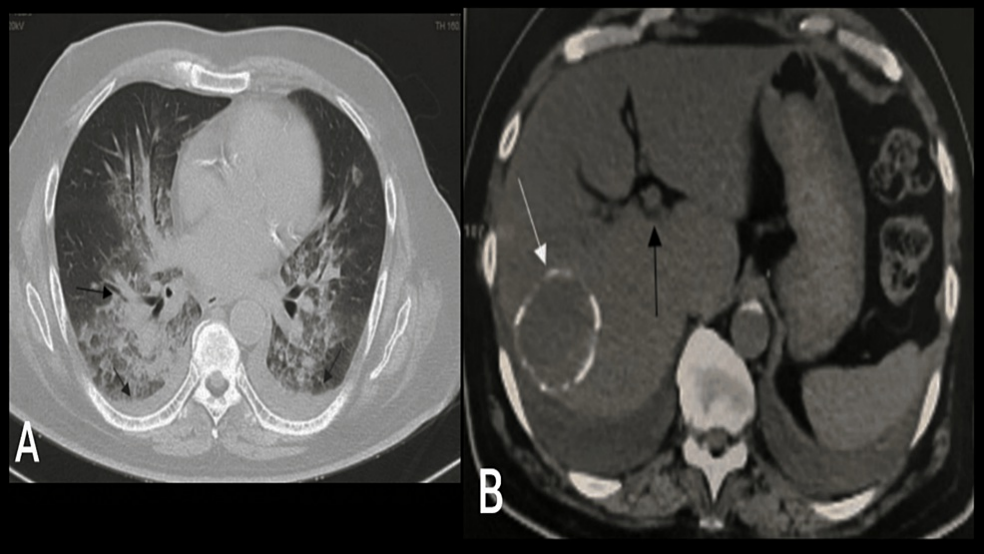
High resolution computed tomography of the chest taken on Day 2 of hospitalization (A) Bilateral perihilar consolidations intermixed with ground glass opacities (black arrows) with bilateral pleural effusion (blue arrows) and pericardial effusion. (B) Abdominal cuts showing unidentified liver lesions (white and black arrows).
